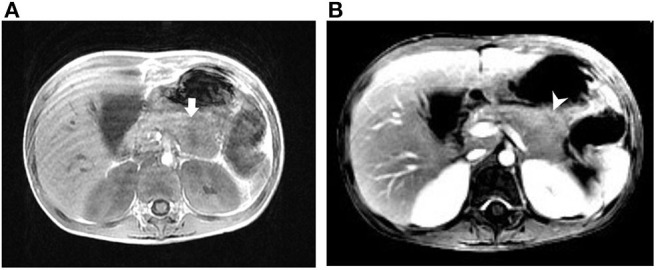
Magnetic resonance imaging of case 1. (A) T1-weighted image (T1WI) showing an ill-defined mass lesion at the pancreatic body. (B) T1WI with contrast showing the slight enhancement of the pancreatic lesion with central hyposignal. Arrow (white), pancreatic mass; arrowhead (white), central hyposignal of the lesion.
